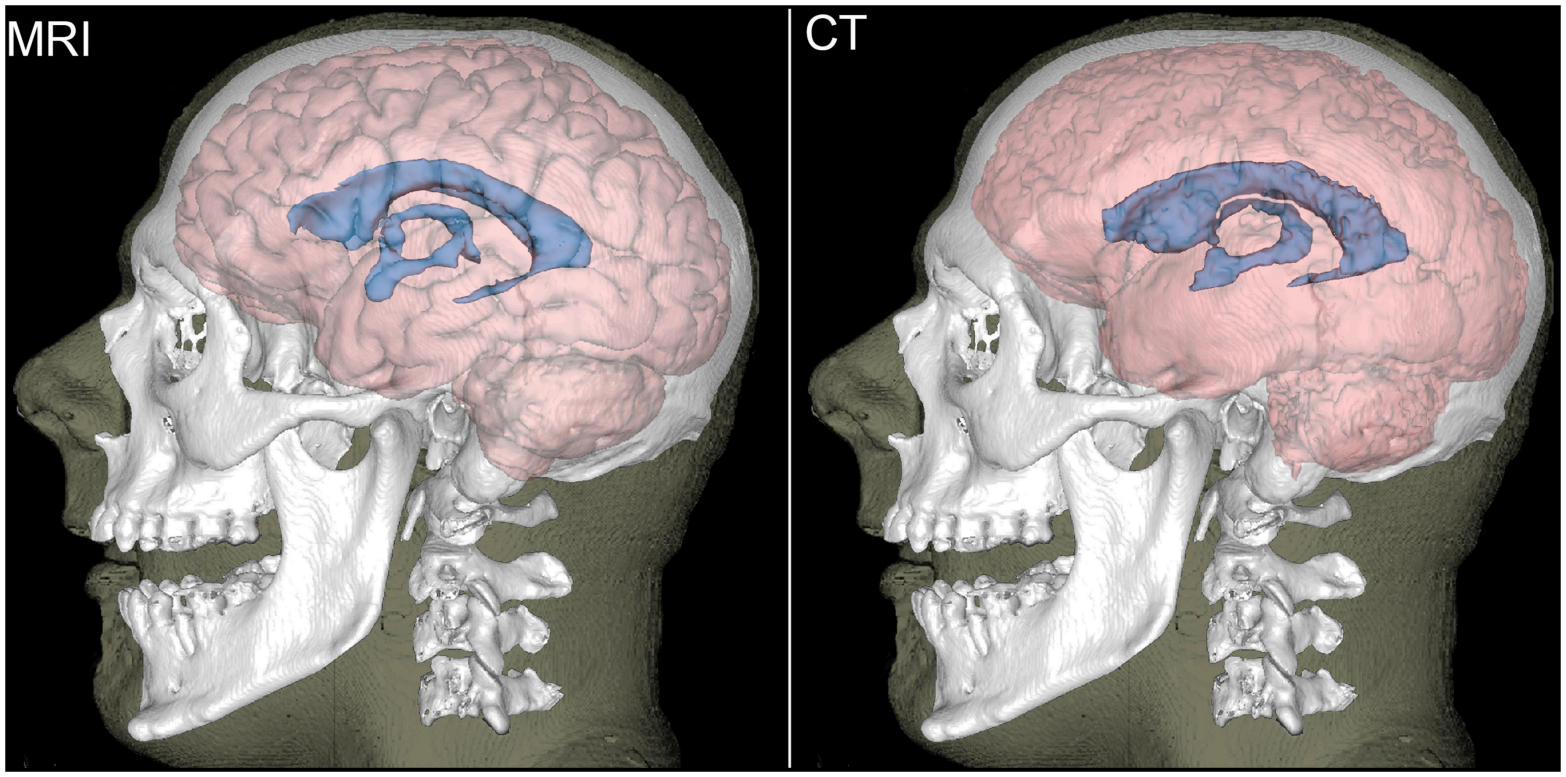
Reconstructions of the brain (light red), ventricular CSF (blue), bones (white), and skin (translucent) for a representative participant. The brain and ventricular CSF are based on MRI (left) and on CT (right). Bones and the skin surface were reconstructed from CT.
